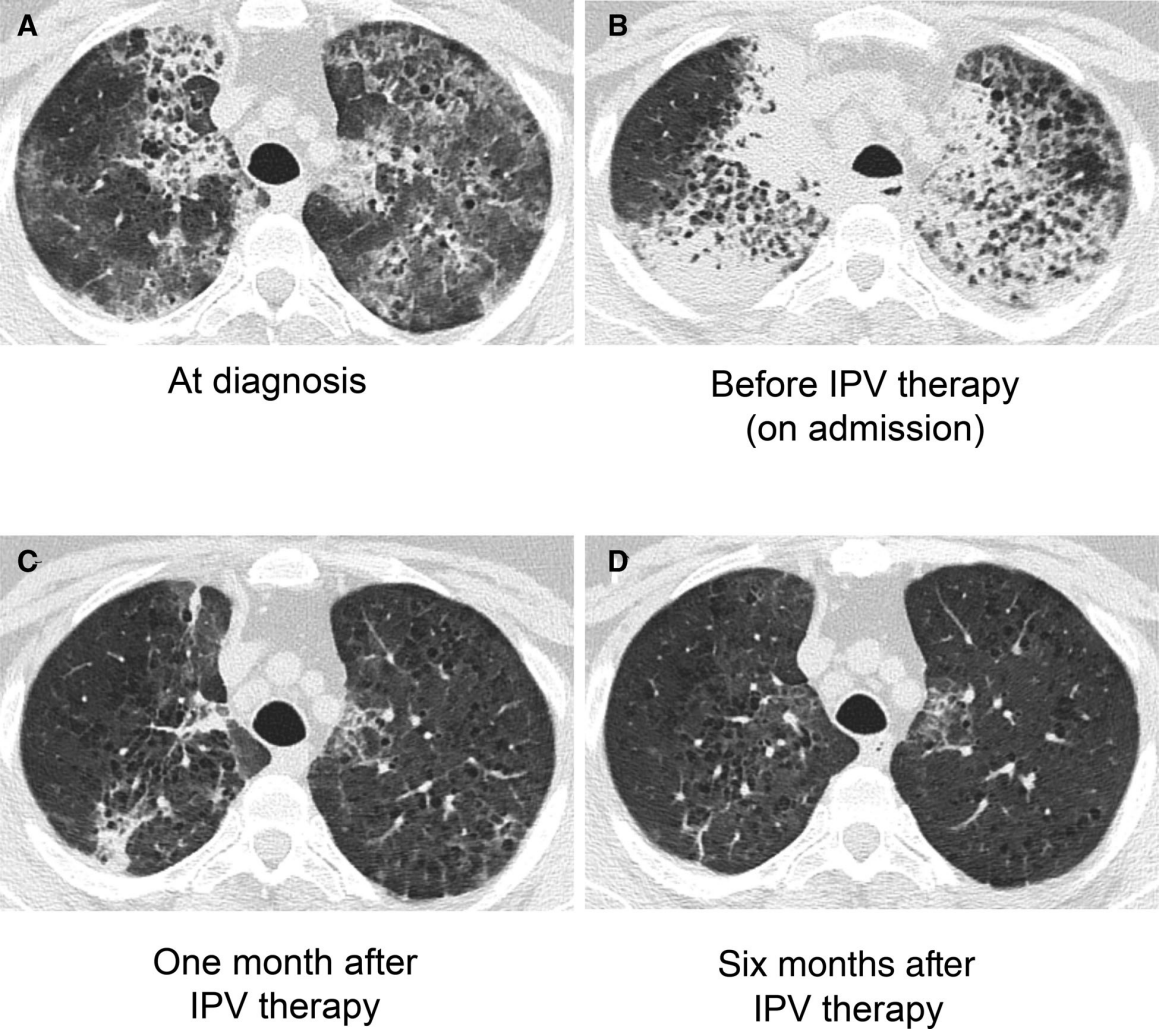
Key imaging. Computed tomography (CT) images at diagnosis (A), before intrapulmonary percussive ventilation (IPV) therapy (on admission; B), one month after IPV therapy (C), and six months after IPV therapy (D) are shown.
